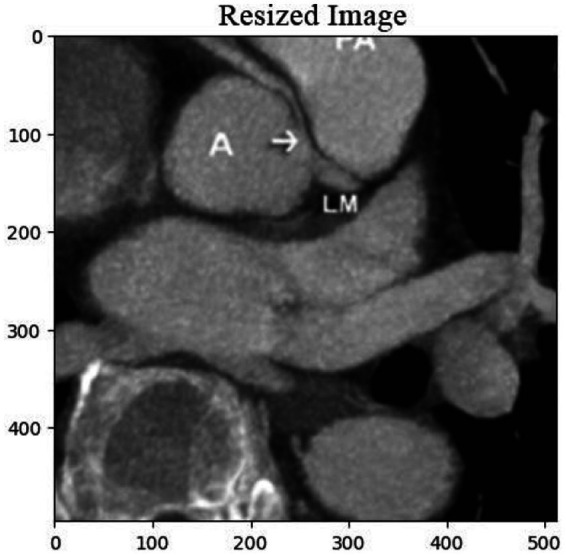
Resized cardiac CT image with dimensions of 64×64 pixels, prepared for further image analysis and feature extraction. This step ensures uniformity across the dataset for optimal processing.
